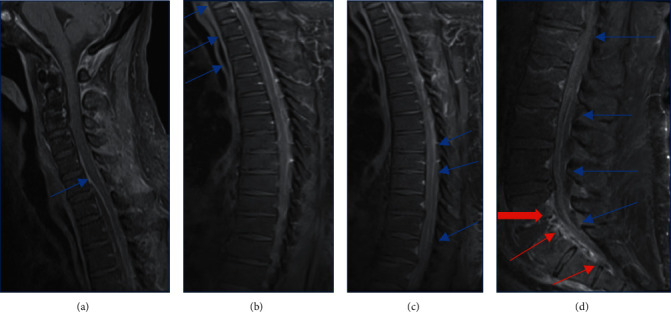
T1-weighted MRI scans: (a) anterior epidural rim enhancing collection extending from C6-T4 (below the image field). The starting point of the abscess is marked by a blue arrow. There is posterior displacement of the spinal cord with significant narrowing of the spinal canal. (b) Extension of the anterior epidural abscess from the cervical spine to the T4 level (blues arrows). There is a mass effect and flattening of an anterior aspect of the spinal cord. (c) Posterior epidural minimally enhancing collection extending from T7-T8 level to L5-S1. (d) The poster epidural collection extending from the thoracic spine to L5-S1 (blue arrows). L5-S1 osteomyelitis-discitis (thick red arrow) and anterior epidural abscess extending from the same level to the lower sacrum.
